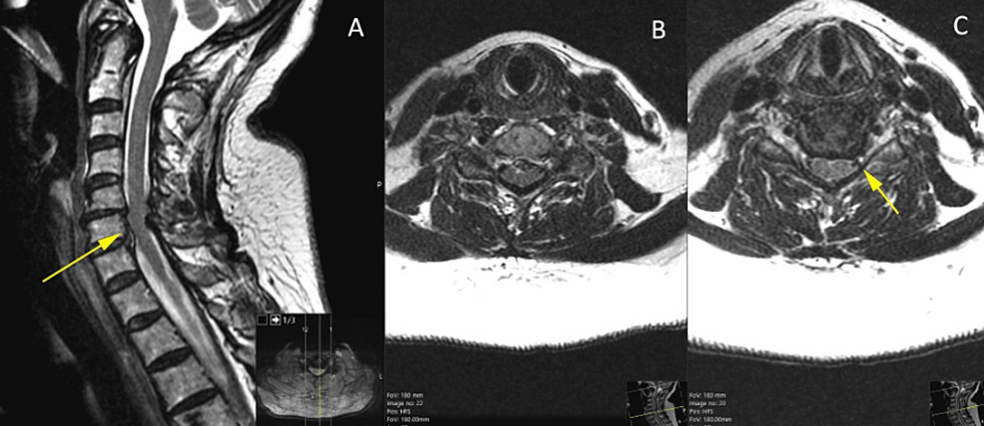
Pre-operative MRI-T2 image Pre-operative MRI-T2 demonstrating central spinal stenosis (anterior-posterior diameter 6 mm) at C5-7 resulting from disc-osteophyte complexes and ligamentum flavum hypertrophy. A. Sagittal view: yellow arrow indicates the area of maximal compression; B. Axial view at the level of maximal cord compression; and C. Axial view: yellow arrow indicates left neuroforaminal stenosis at C5-6.
